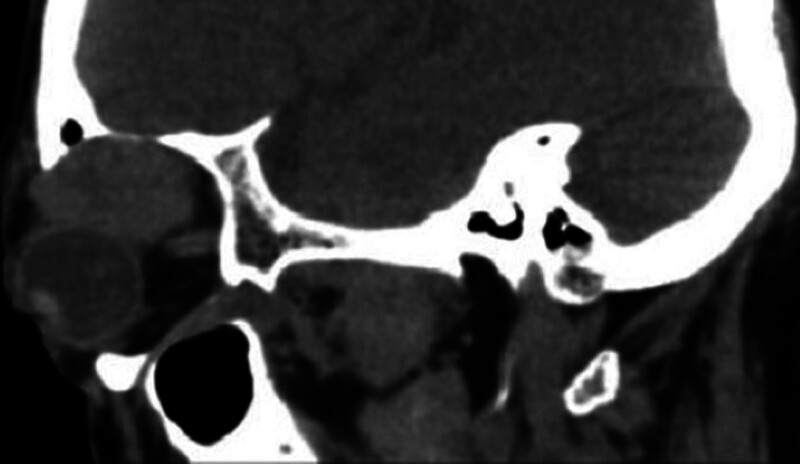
CT scan suggested an oval soft tissue mass (approximately 3.3 × 2.3 × 2.4cm in size, with clear boundary, uniform density, enlarged lacrimal fossa, and no bone destruction) in the extrapyramidal space. CT = computed tomography.
